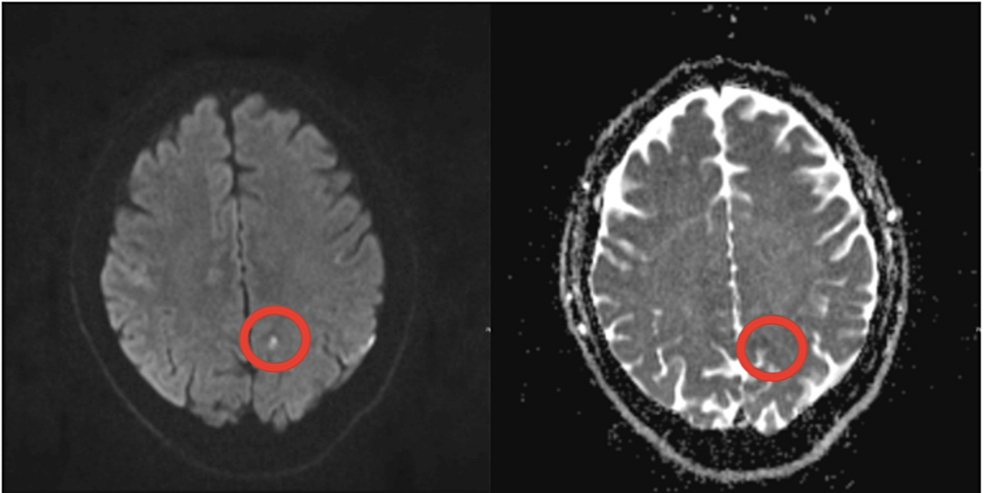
Axial B1000 DWI MRI (left) and corresponding ADC map (right) reveal a tiny less than 5 mm diameter of acute diffusion restriction in the left posterior cerebral hemisphere most in keeping with a tiny acute focus of infarction (labelled) DWI: Diffusion-weighted imaging; ADC: apparent diffusion coefficient
